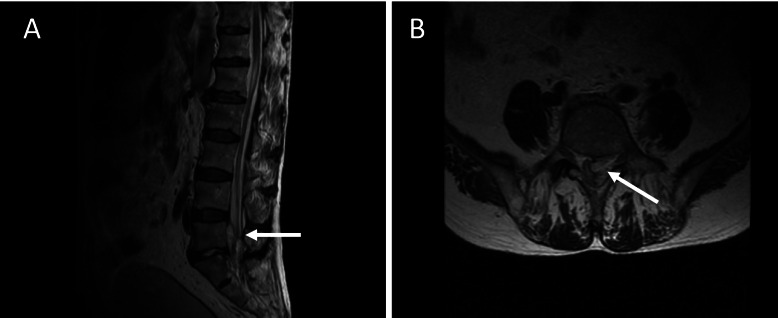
Lumbar magnetic resonance imaging. a Sagittal lumber T2-weighted images show a high signal intensity at the L5-S1 vertebral disc, indicating an epidural abscess (arrow). b Axial T2-weighted images reveal abscess at the L5 disc (arrow)
